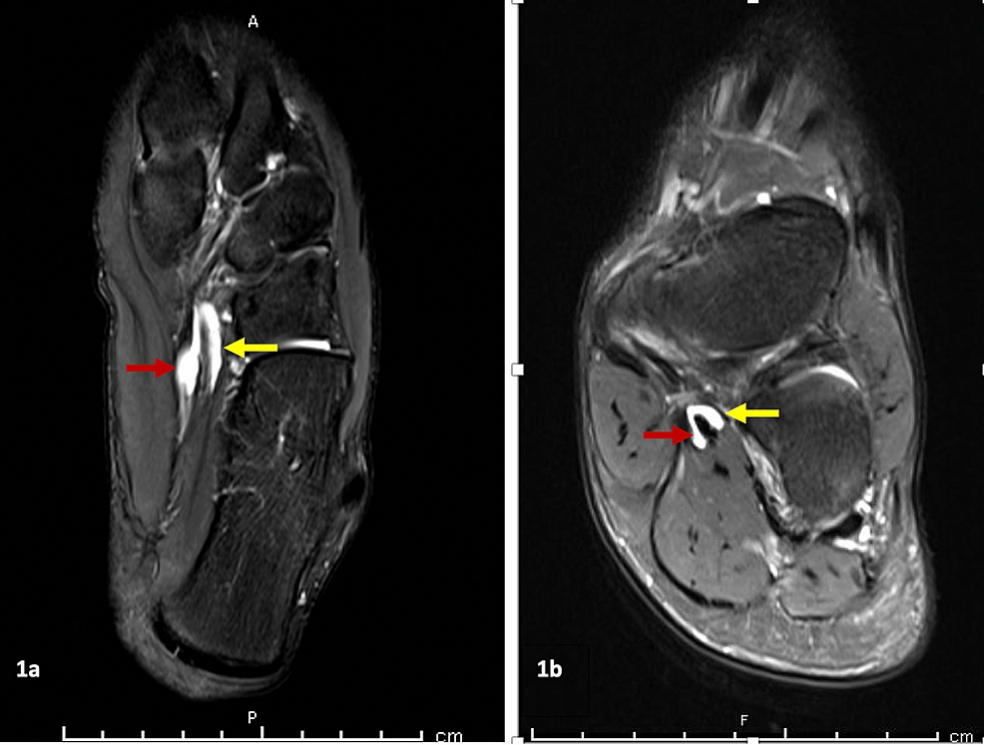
Axial (1a) and Coronal (1b) T2-weighted MRI of Peritendinous Fluid around the FHL (yellow arrow) and the FDL (red arrow) Sheaths. FHL = flexor hallicus longus FDL = flexor digitorum longus
